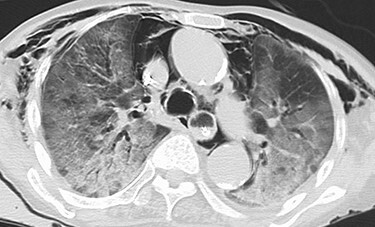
Chest CT showing mediastinal and subcutaneous emphysema.
